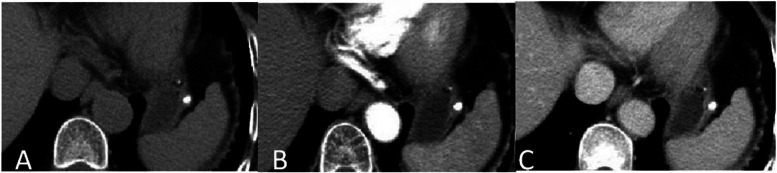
With multiple stromal tumors in the gastric fundus. A Unenhanced CT scan showed two nodular calcifications in the gastric fundus, with a smaller one located in the submucosal layer and a larger one located in the musculoserosal layer. CT scan in the arterial phase (B) and portal vein phase (C) showed no heterogeneous enhancement around the lesion
